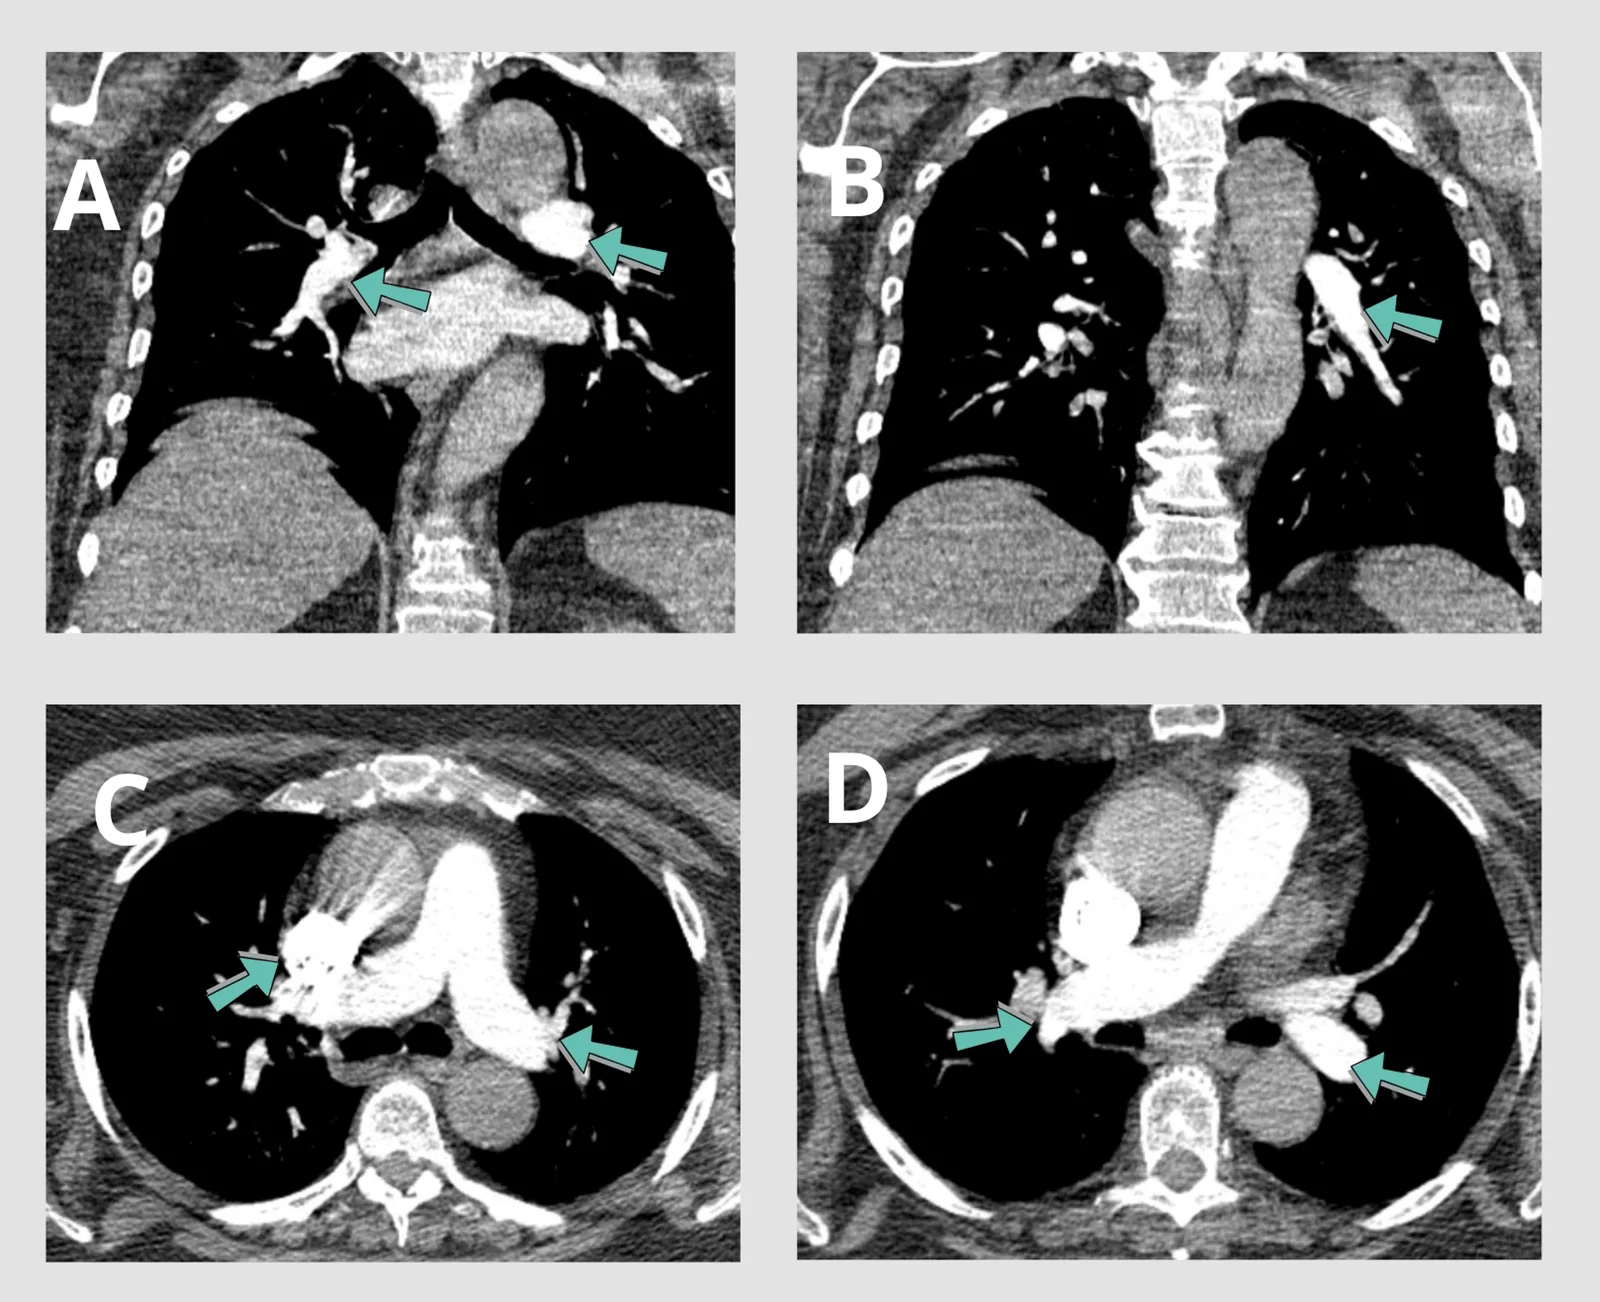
Six-month follow-up computed tomography pulmonary angiography after anticoagulation Follow-up contrast-enhanced computed tomography pulmonary angiography of the chest performed after six months of anticoagulation. A and B (coronal views): Multiplanar coronal reconstructions showing adequate opacification and patency of the right and left pulmonary arteries (arrows). C and D (axial views): Transverse sections at the level of the main pulmonary artery and its main branches. No intraluminal filling defects are identified in any projection, confirming the complete resolution of pulmonary embolism (arrows).
